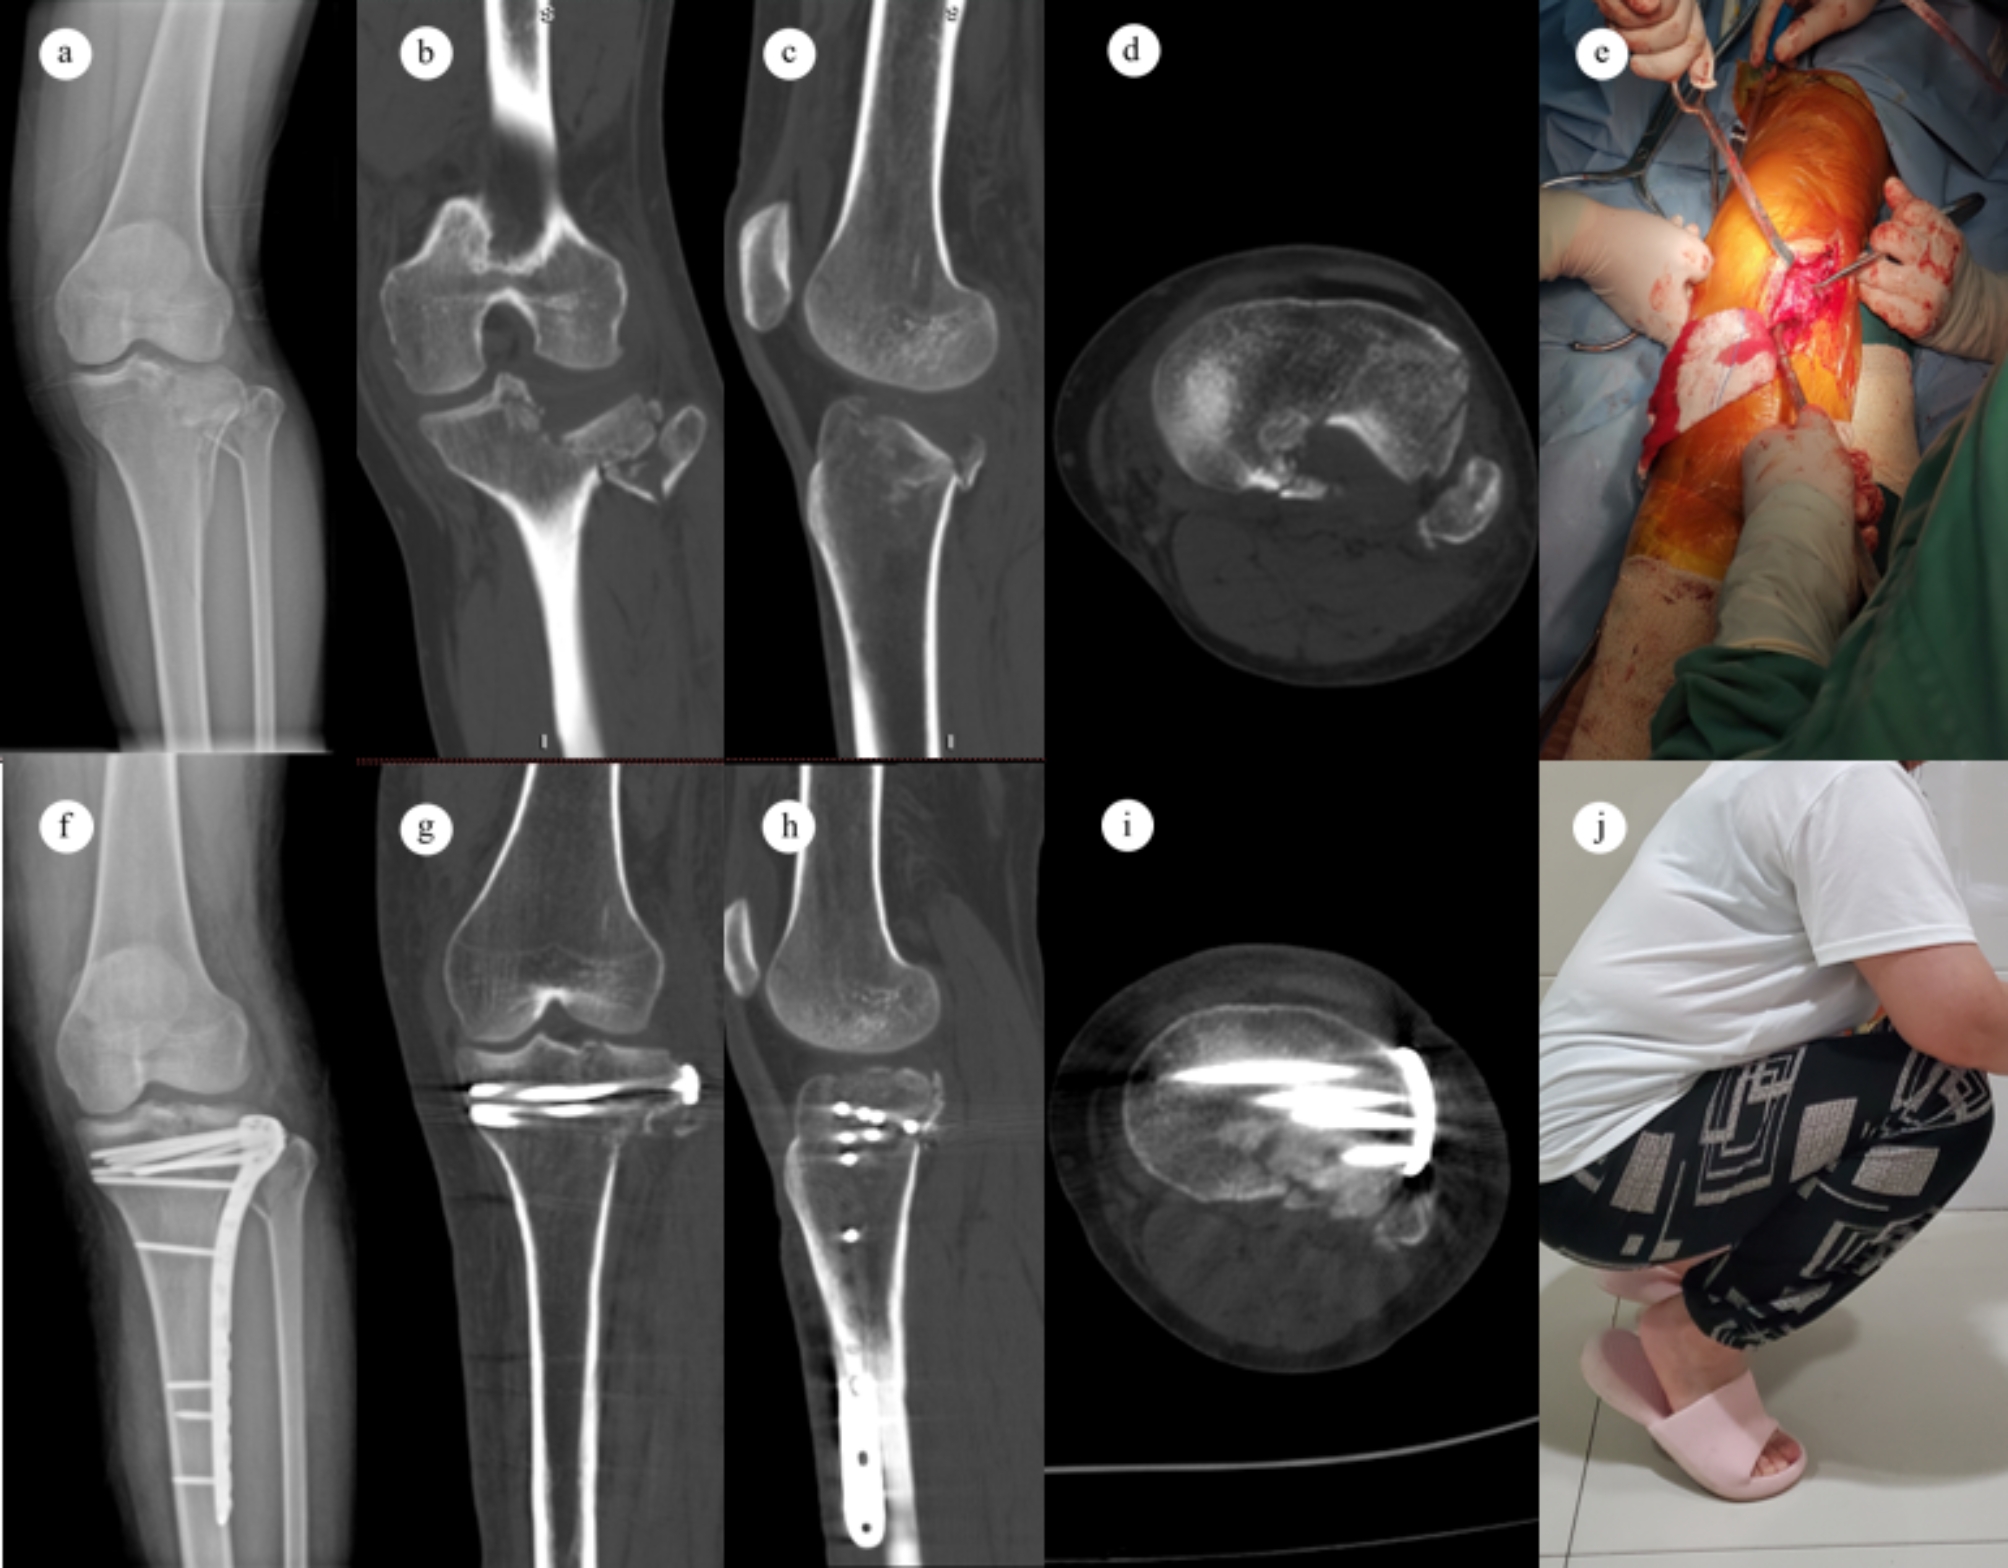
A case of type II tibial plateau fracture, female, 64 years old. a-d Preoperative X-ray and CT showed a significant depressed fragment in posterolateral plateau. e Intraoperative image showed a cortex window was created on the anterolateral plateau and the depressed posterolateral fragments were elevated through the window with a metal bone tamp. f-i Postoperative X-ray and CT showed that the fracture was anatomically reduced. j This patient’s 6-months follow-up
